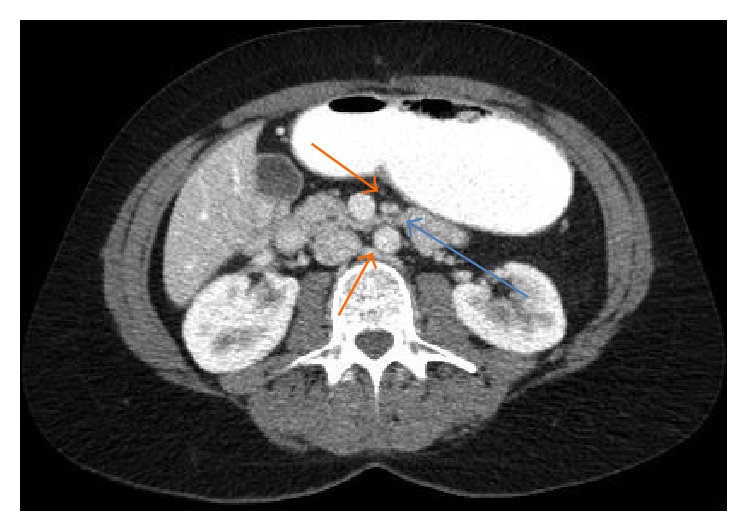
Duodenal compression on CT scan (blue arrow) between the aorta and superior mesenteric artery (orange arrows) (Case Report Two).
